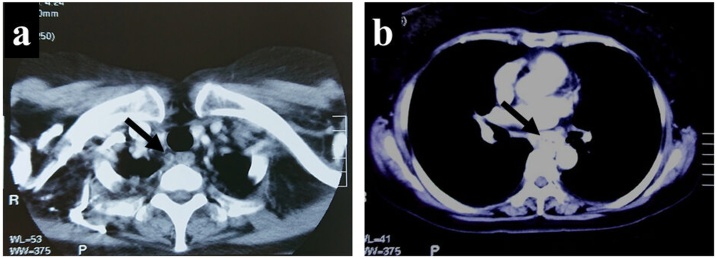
a, b: A CT scan revealed the upper mediastinal lymph node swelling and thickening of the esophageal wall.
